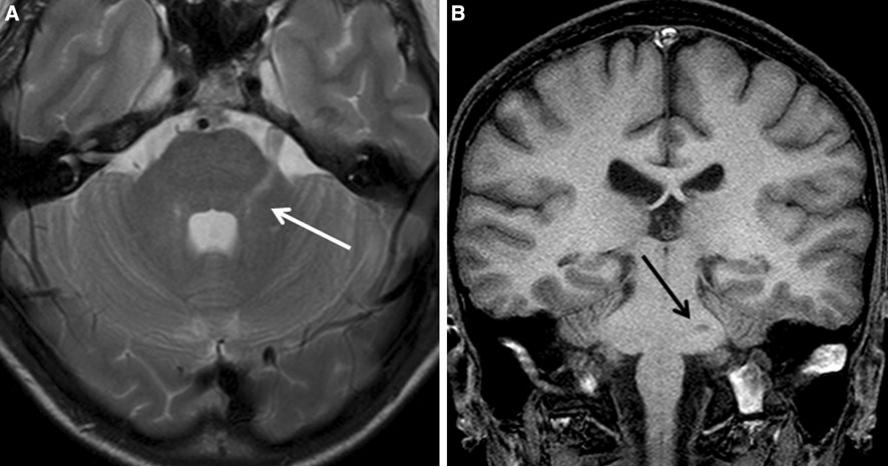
This figure represents the first MRI (without gadolinium) study performed on the patient: axial T2 (a) and coronal T1-weighted images (b) showing T2 hyperintense signals located at the territory of the* left* trigeminal root inlet and main sensory nucleus in the brainstem, which is hypointense in T1-weighted image coronal section (b)
